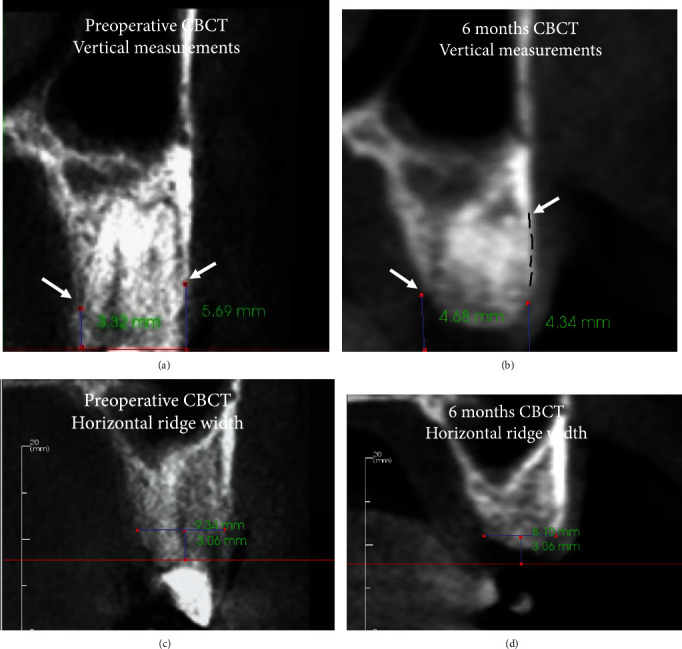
(a and c) Preoperative CBCT and (b and d) 6 months CBCT views were used for linear and horizontal measurements. Six months cross-sectional CBCT showed buccal augmentation and complete infill (delineated in dashed line) to the crest of the buccal defect (b, arrowed). Vertical measurements were taken from the CEJ to the buccal, or lingual alveolar crest (arrowed). (a and b) The preoperative BRH was 5.69 mm, and the postoperative BRH was 4.34 mm. (a and b) The preoperative LRH was 3.32 mm and postoperatively this measured 4.34 mm. (c and d) The preoperative HRW was 9.34 mm and reduced slightly to 8.10 mm postoperatively.
